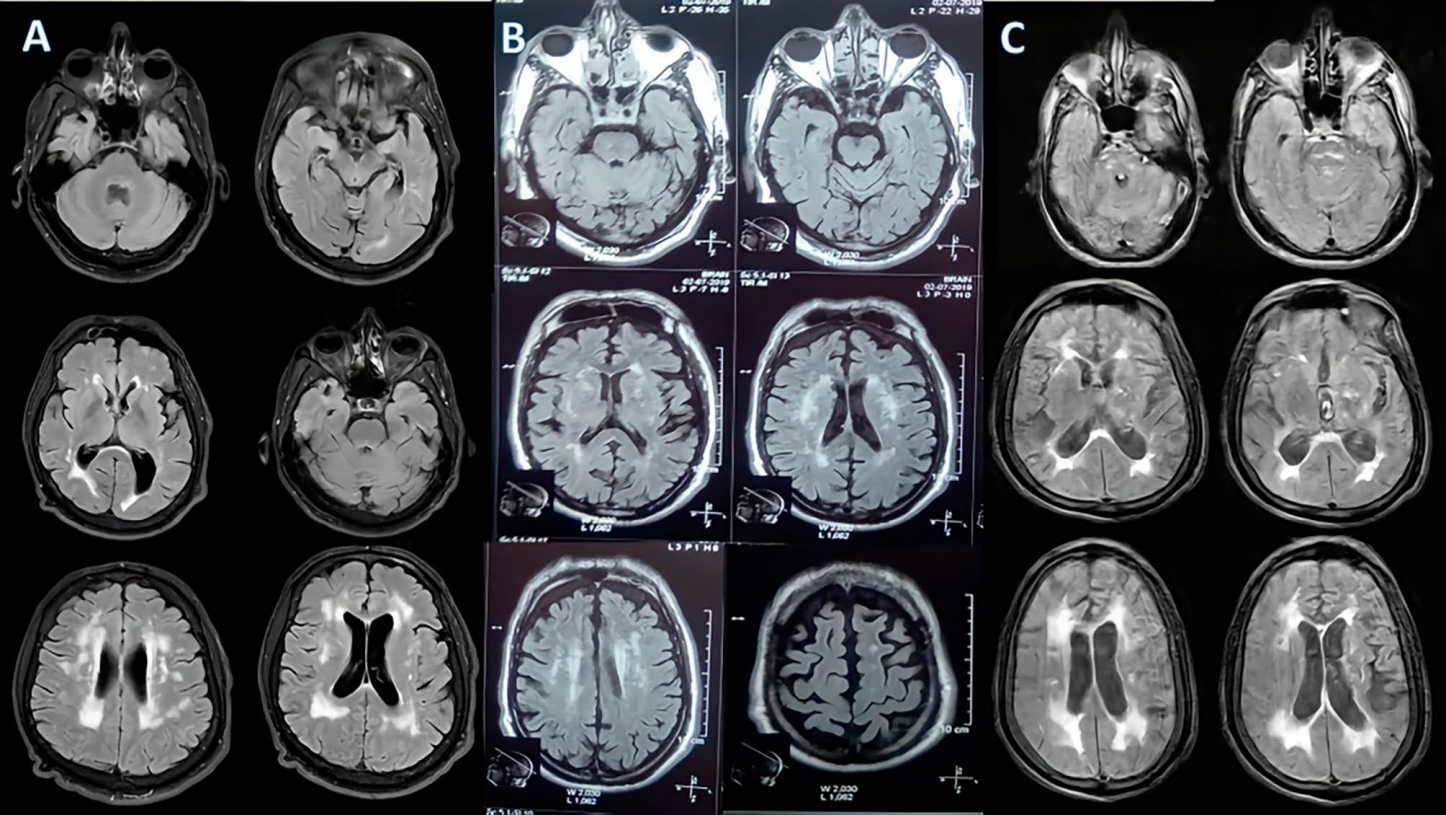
Examples of three patterns of white matter hyperintensities demonstrated in Brain MRI FLAIR: (A) bilateral cerebral periventricular bright signal foci and confluent patches, (B) bilateral cerebral periventricular and basal ganglia signal abnormalities, (C) bilateral cerebral periventricular, basal ganglia and pontine hyperintensities
